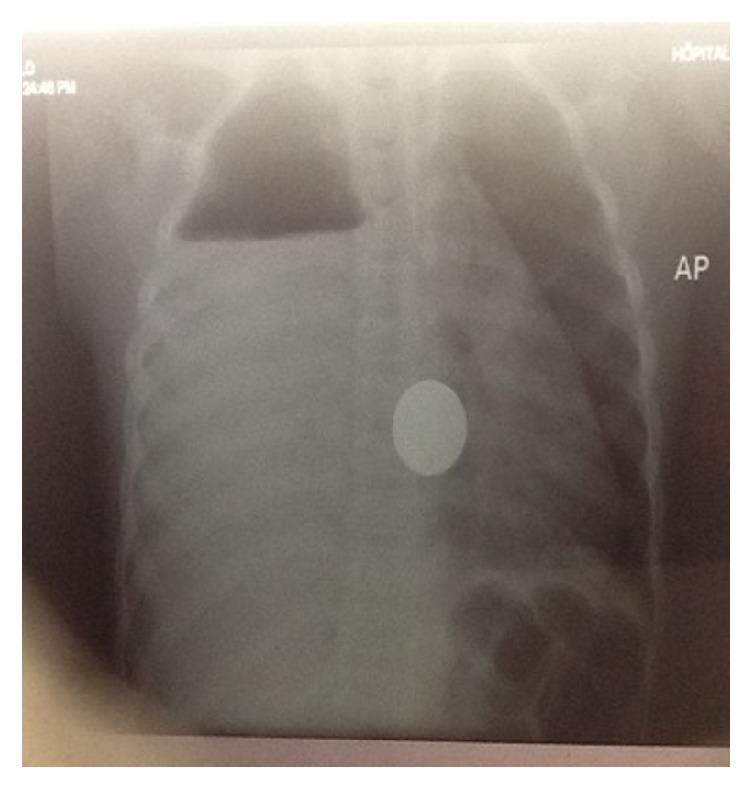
Chest X-ray of the 6 Y/O girl after the incident. Massive pleural effusion and air fluid level; foreign body in the right chest at the anatomical site of the esophagus and radiological signs of perforation.
